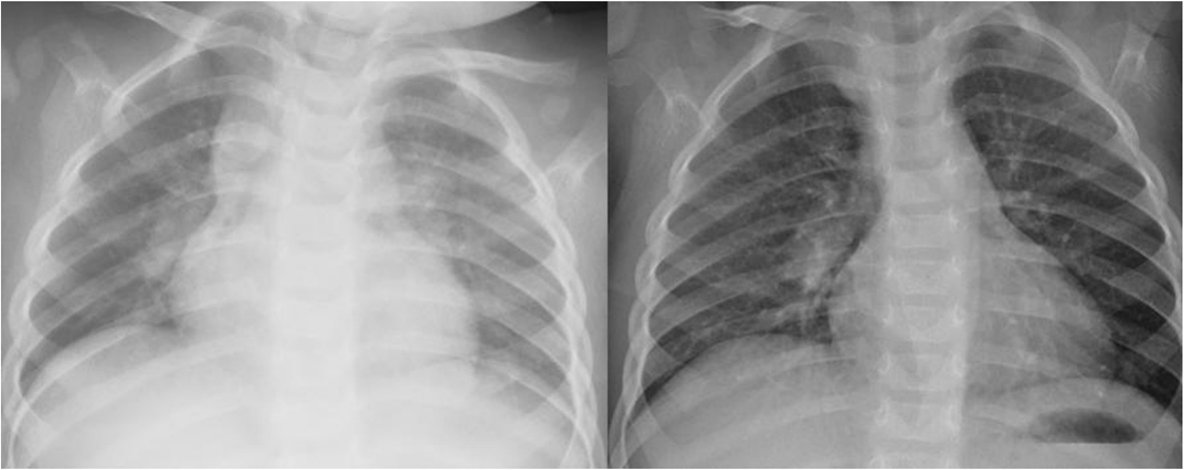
chest x-rays findings. Evidence of right mediastinal enlargement (*figure on the left*) on first control, with disappearance on second evaluation after prednisone course (*figure on the right*)
